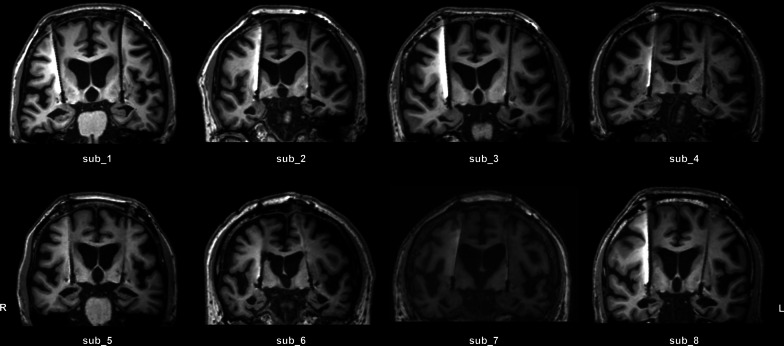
Post-operative MRI showed the NBM lead location for each subject (*n* = 8)
